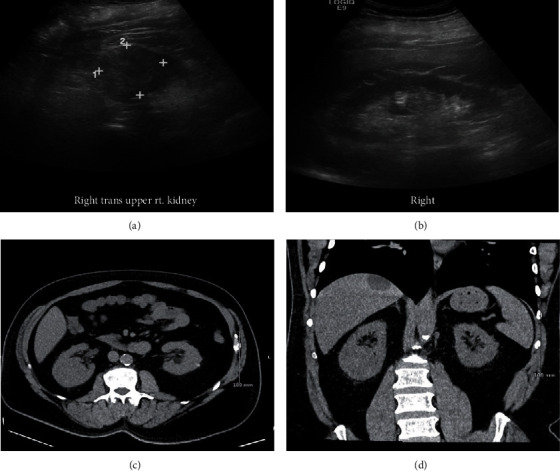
Ultrasound findings: transaxial (a) and long axis (b) views show the right kidney containing a solid, heterogeneous isoechoic mass in the midpole. CT abdomen without IV contrasts coronal (c) and axial (d) views, respectively: a solid isodense mass of the mid to inferior pole of the right kidney protrudes into the renal sinus. There is minimal deformity of the renal contour by the lesion. An incidental cystic lesion of the liver is seen.
